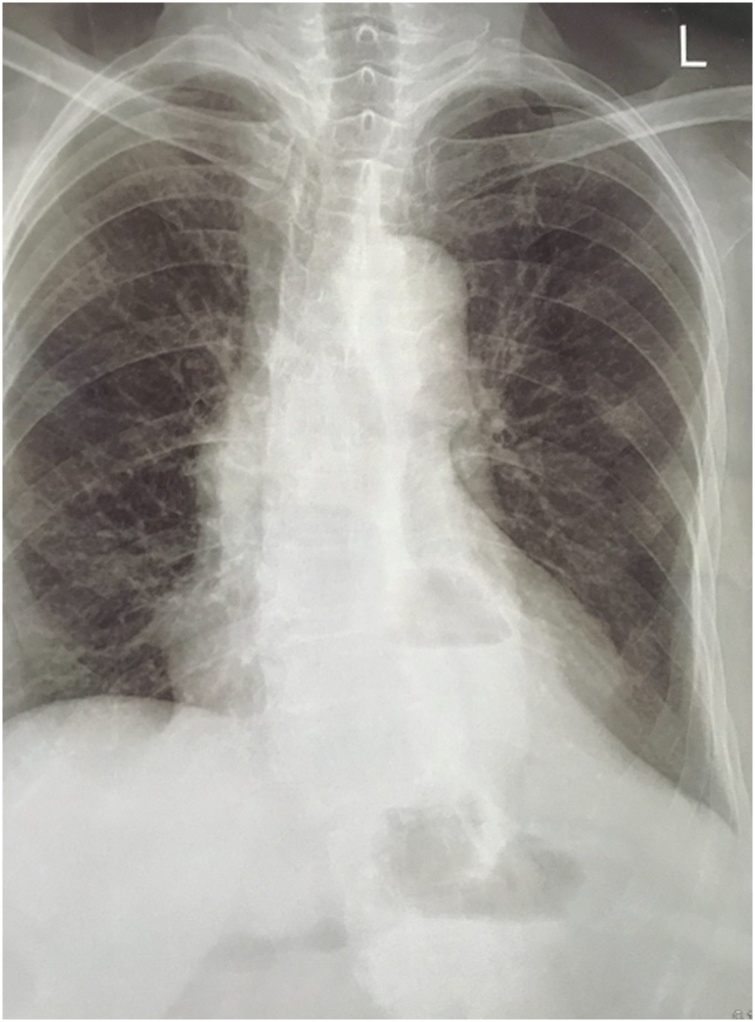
X-ray at six months follow up.
